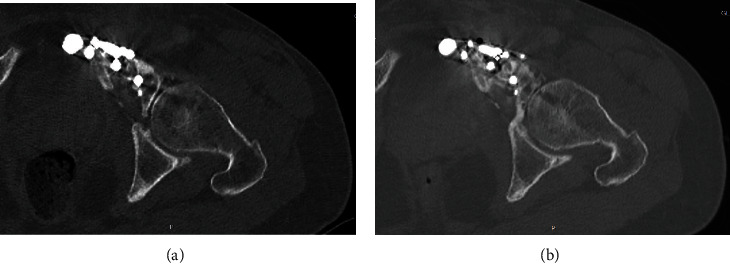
Axial view of a CT scan at 6 months (a) and at 30 months of follow-up (b) showing the healing of the osteotomy and the progression of osteoarthritis in the articular portion of the recycled autograft.
